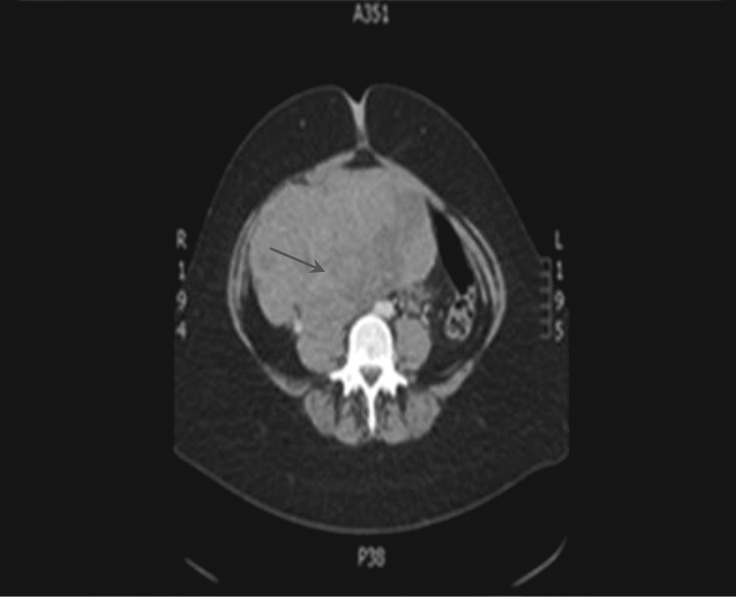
CT abdomen showing large right adnexal mass (red arrow).
